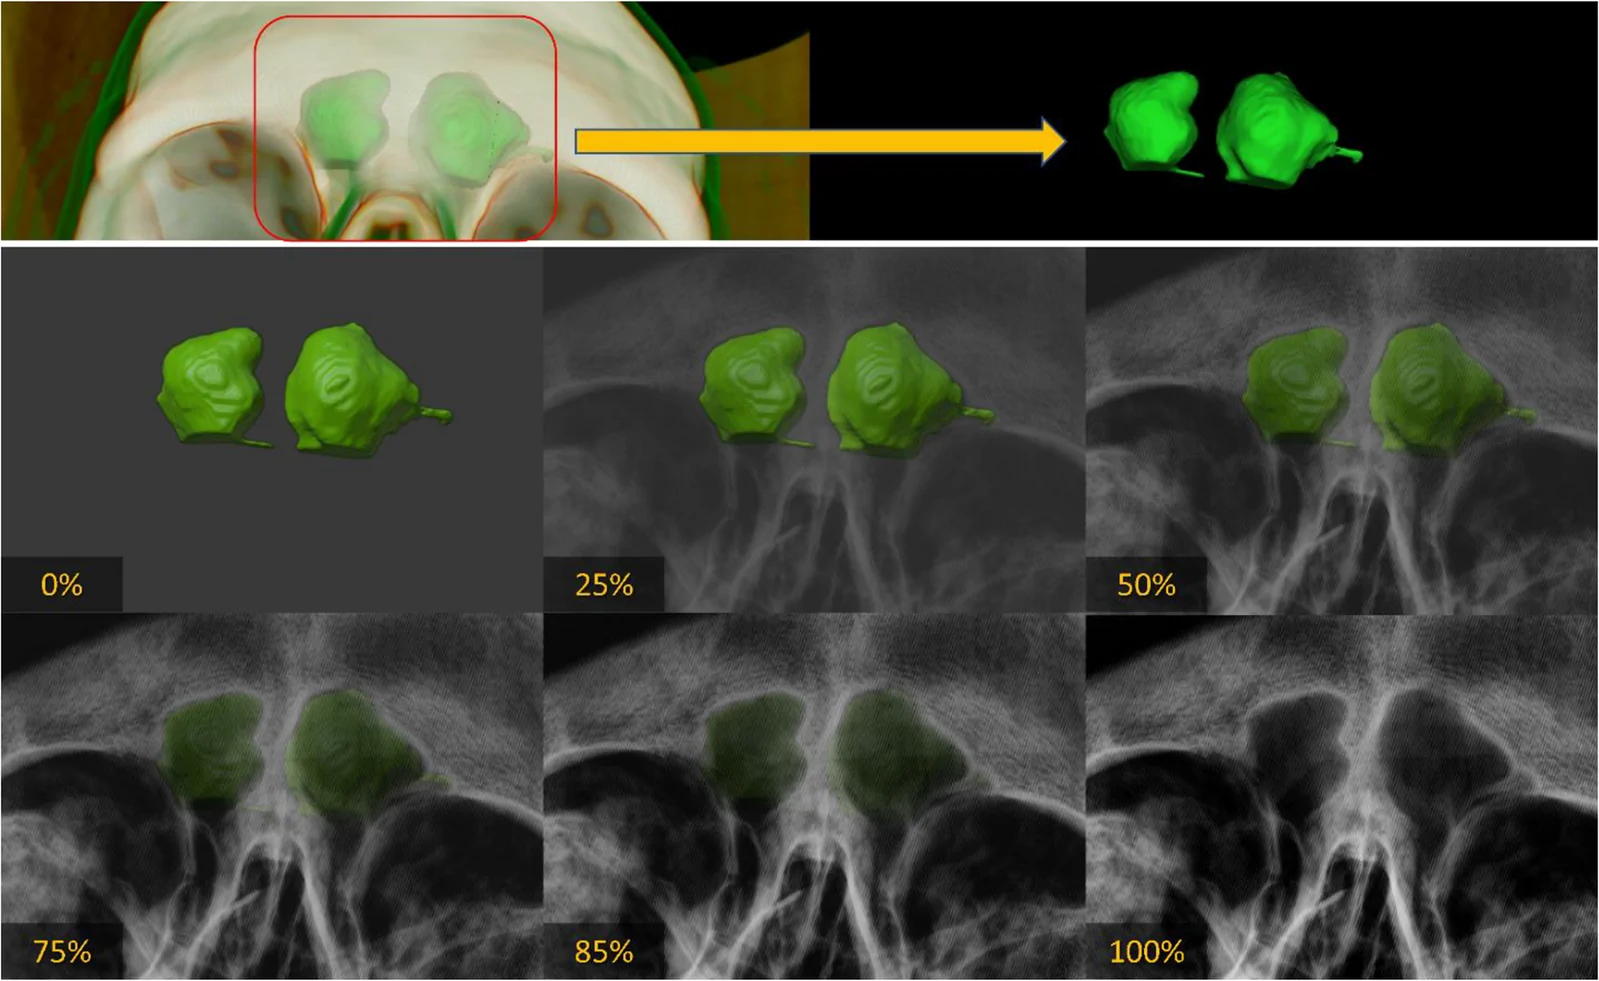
Process generating a digital 3D model of the frontal sinus from the reference CT. Below, this file was adjusted for scale and rotation on the PM radiograph, demonstrating morphological compatibility between the two records. The degree of radiographic image opacity is indicated in each segment
